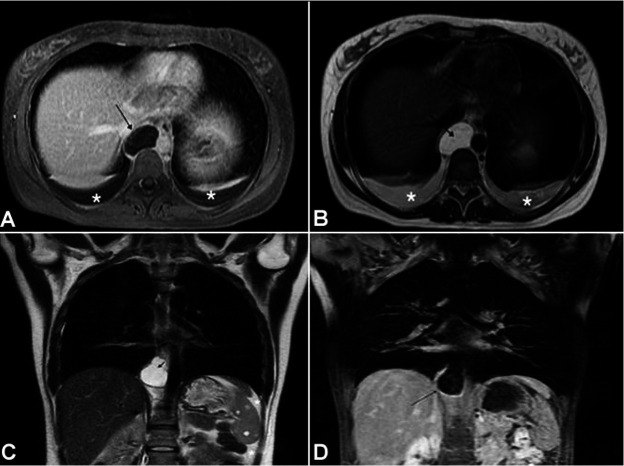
Axial MRI sections of the chest showing a cystic image in the topography of the thoracic duct (arrow) characterized by A – a low signal in the T1-weighted sequence; B – a high signal in the T2-weighted sequence; associated with C – small pleural effusion and ascites (asterisks); the cyst is also evident (arrow) in the coronal section on the T2-weighted sequence, and D – without enhancement in the T1-weighted sequence after contrast injection.
